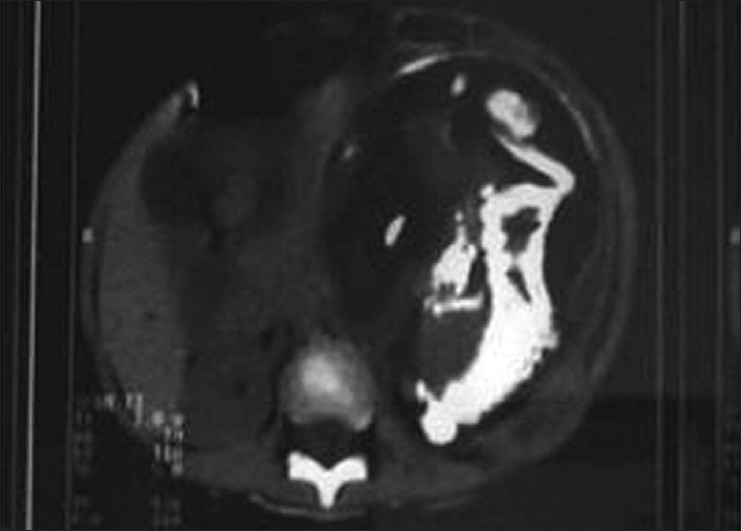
CT scan showing large retroperitoneal mass with a variable consistency
